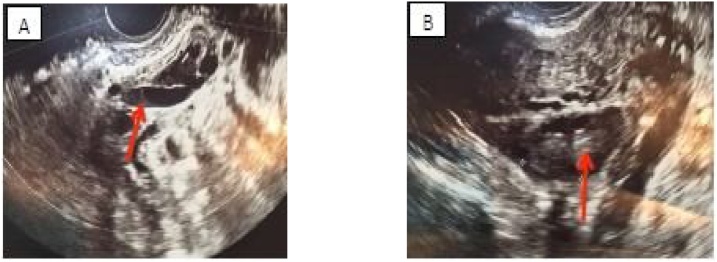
(A & B): Trans-Abdominal Ultrasound of the left ovary showing a dilated left ovarian vein.
